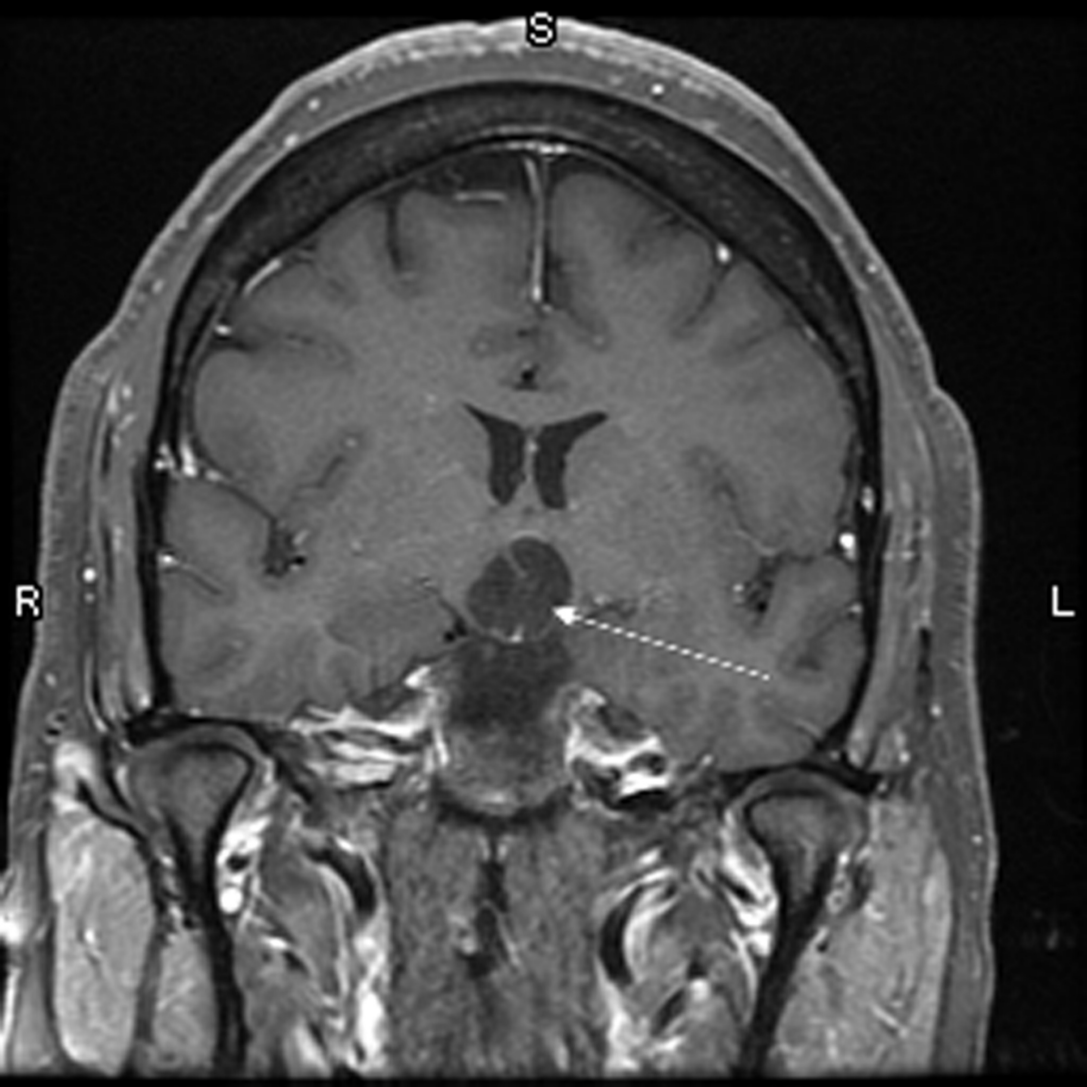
Coronal T1 with contrast Preoperative brain MRI showing a multi-locular suprasellar cyst (solid white arrow) measuring approximately 1.6 x 1.8 x 2.4 cm
